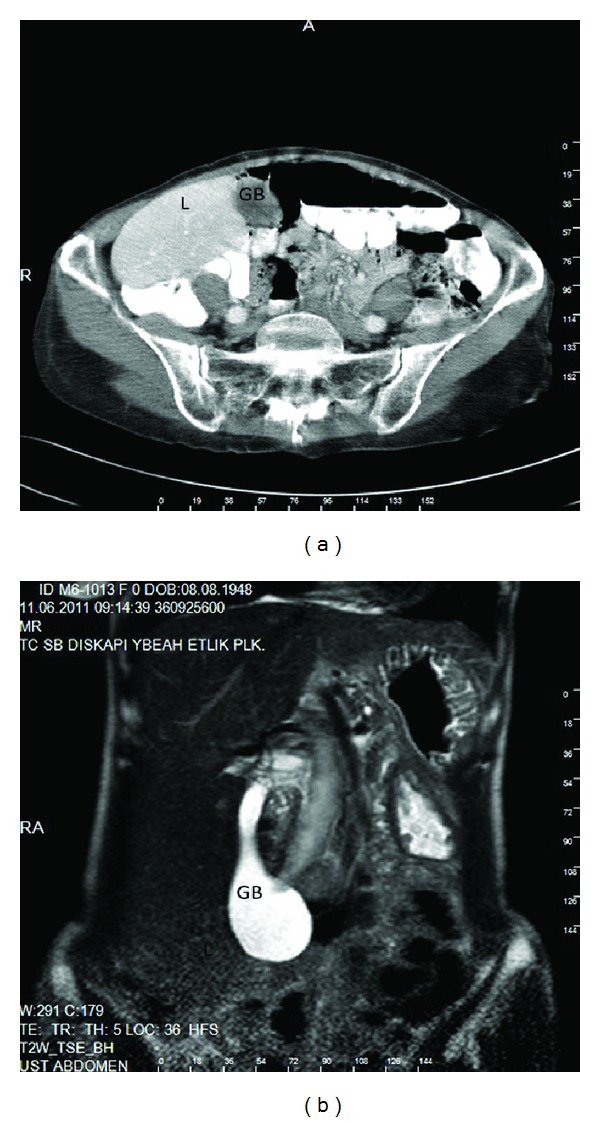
The GB was located in the right lower quadrant (ptosis). ((a) CT and (b) MRI findings) L: liver and GB: gallbladder.
